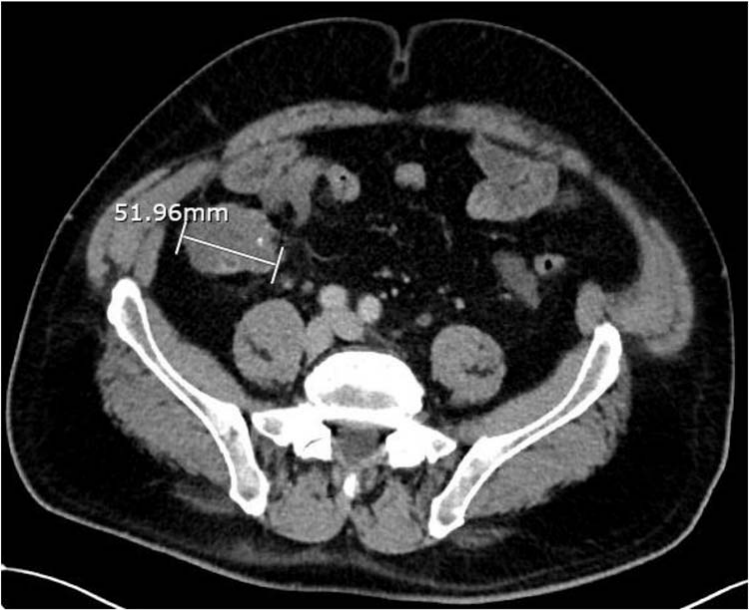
Axial CT abdomen with contrast demonstrating an appendiceal mass of maximum diameter 51.96 mm.
